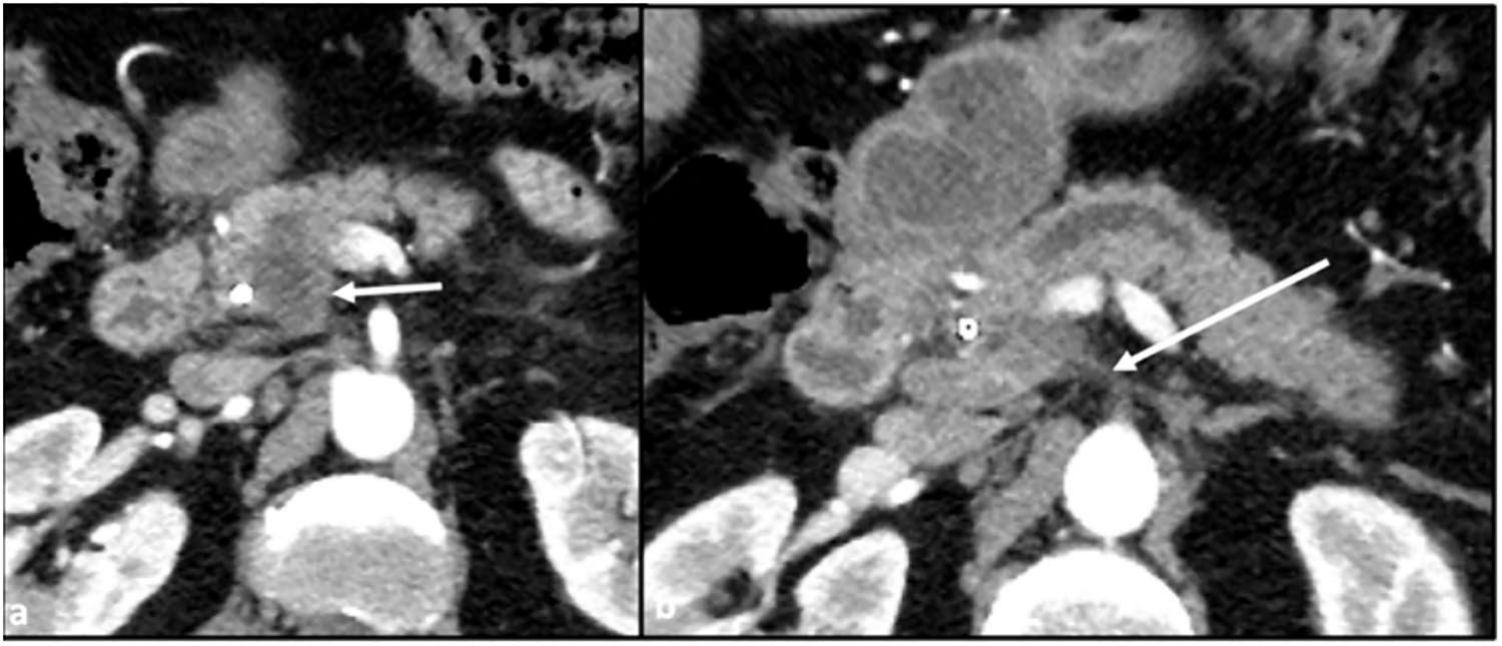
Axial CT sections with contrast in the arterial phase. a Hypodense mass in the posterior pancreatic head (arrow). b Early spread of disease into the superior mesenteric plexus (arrow)
